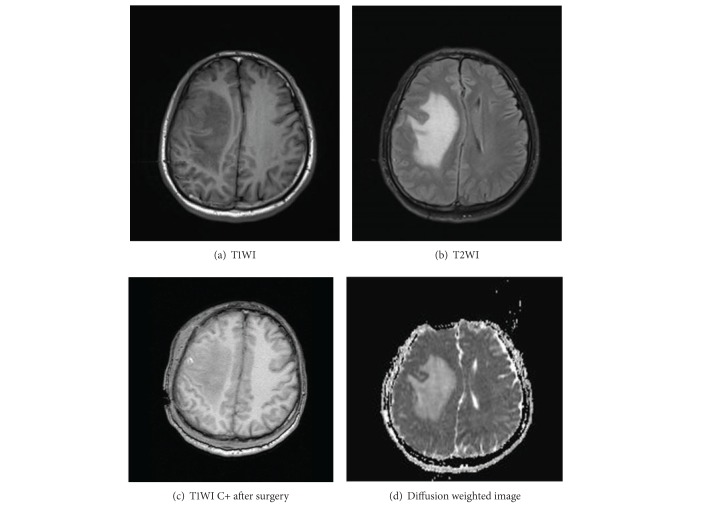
(a) T1WI images shows slightly hypointense lesion on Rt. temporofrontal region with slight midline shift. (b) T2WI shows hyperintense mass by edema surrounded with midline shift. (c) T1WI C+, after surgery, shows isointense lesion. (d) DWI: diffusion weighted image hyperintense lesions with midline shift.
